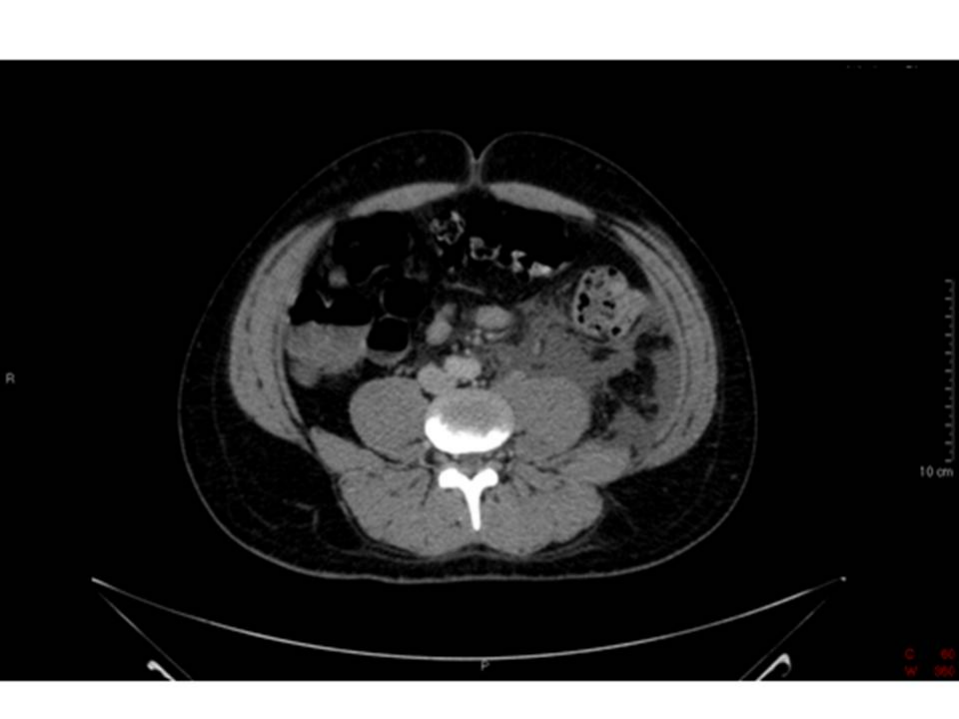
CT Abdomen and Pelvis. Loculated fluid and inflammatory change anterior to the left psoas muscle.
